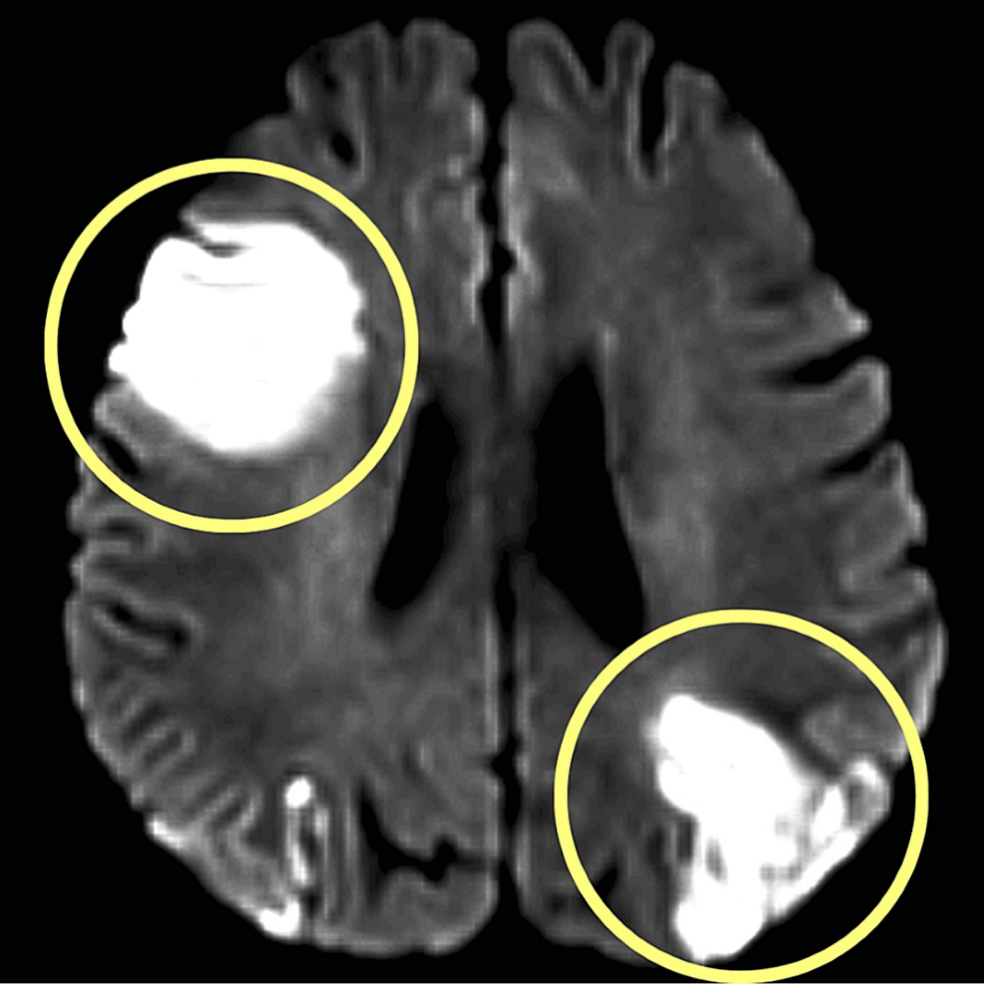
MRI findings. Yellow circles highlight acute infarct in the right frontal lobe, right insular cortex, bilateral parietal lobes, right posterior temporal lobe, and bilateral occipital lobes.
